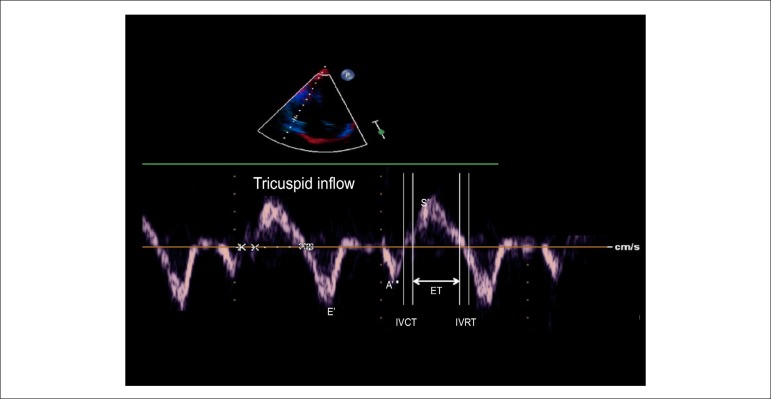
Tissue Doppler imaging performed at tricuspid annulus in apical
